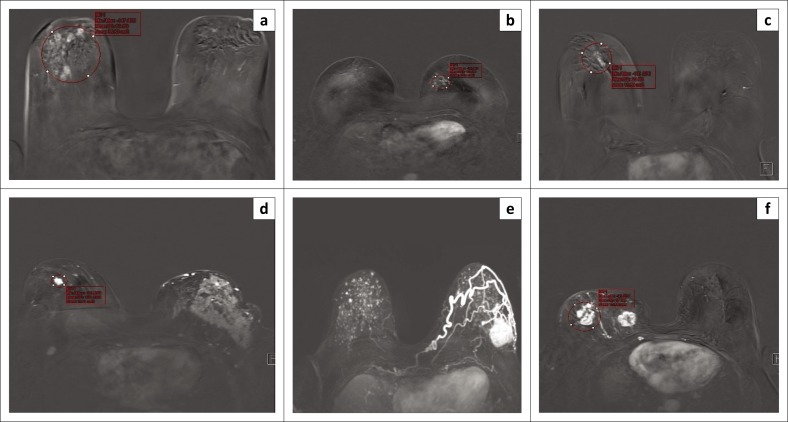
Dynamic contrast-enhanced magnetic resonance imaging enhancement characteristic of various patients with breast cancer: (a) clustered ring, (b) clumped, (c) linear distribution, (d) homogeneous (e) larger homogeneous pattern and (f) multicentric heterogeneous enhancement.
